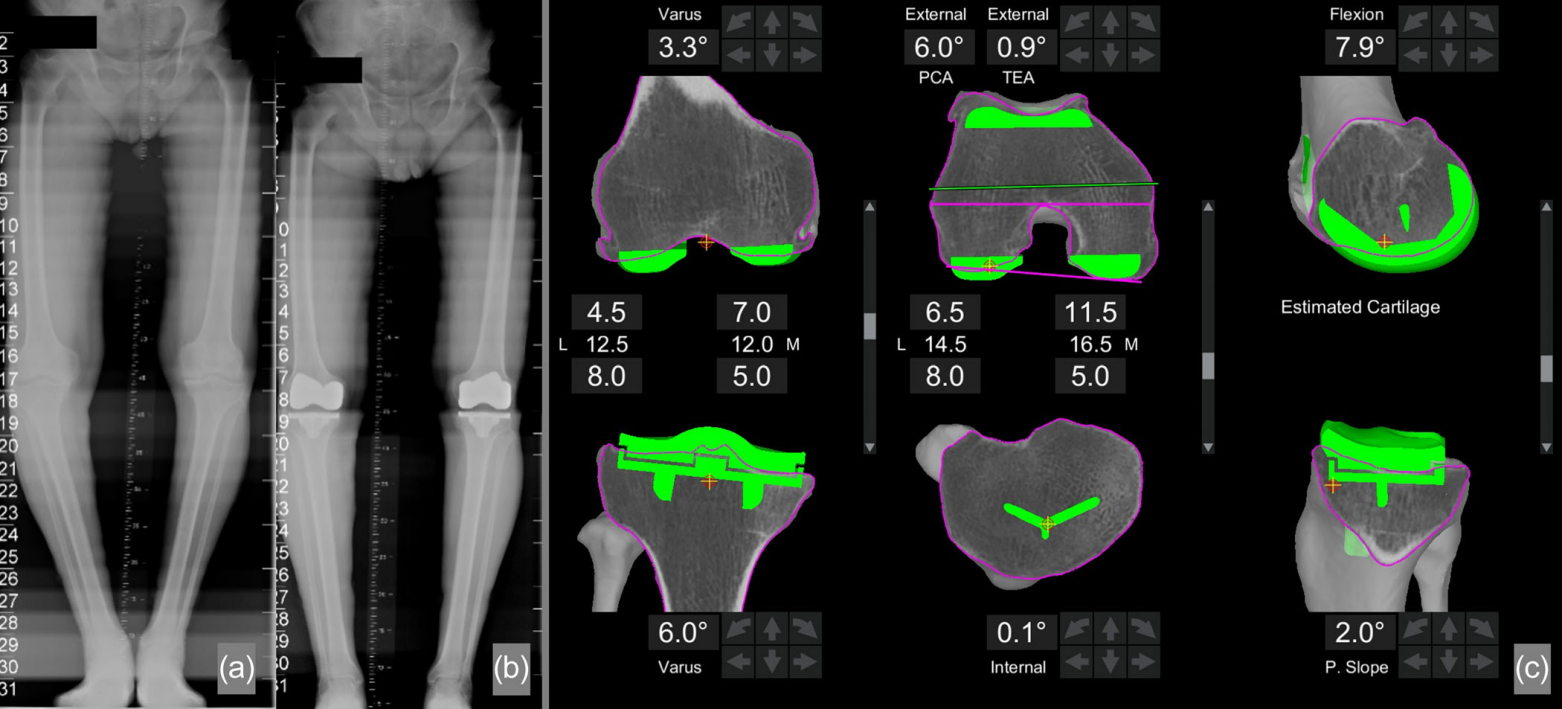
Seventy‐five‐year‐old male patient with a major varus coronal deformity, presenting a preoperative mechanical hip–knee–ankle (mHKA) angle of 161° on the right side, corrected to 171° after total knee arthroplasty (TKA). (a) Preoperative long‐leg standing radiograph; (b) postoperative long‐leg standing radiograph; (c) Intraoperative screenshot of TKA performed with the Mako® robotic system (Stryker ®), showing planning adjustments according to functional knee positioning principles.
